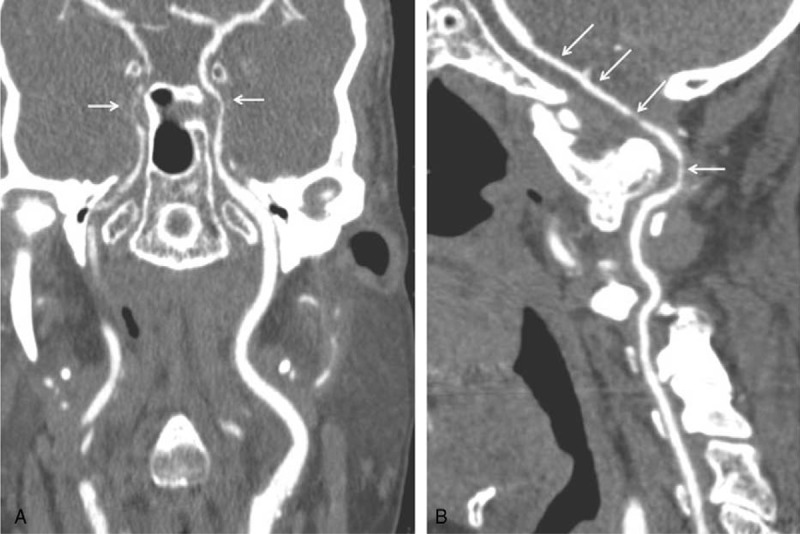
Cranial CT angiography in multiplanar reconstruction. Long, regular, circumferential stenosis of the cavernous portion of both carotid arteries (A). Regular narrowing of the V3 and V4 segments of the vertebral arteries (B).
